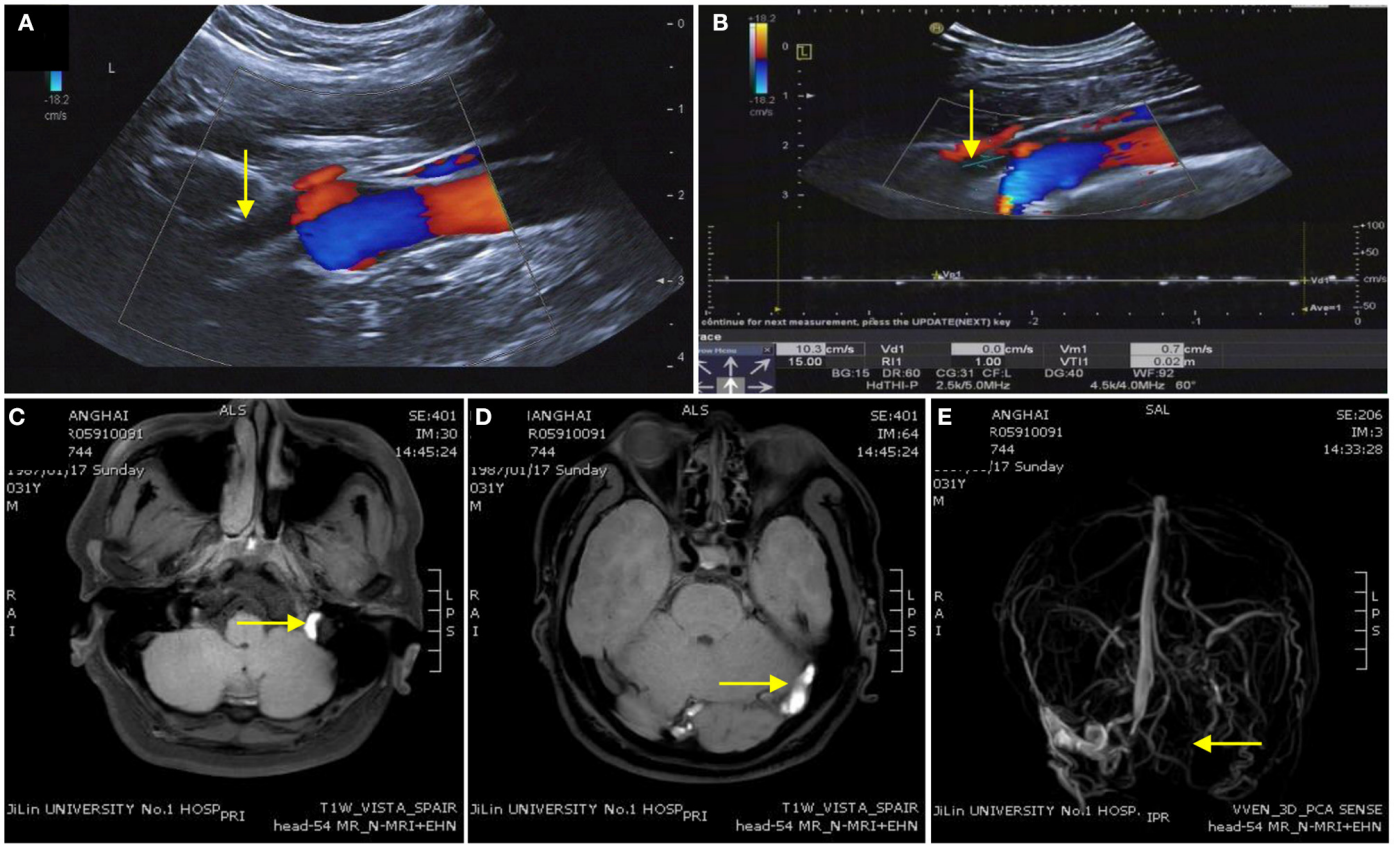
Imaging of a patient with cerebral venous sinus thrombosis. (A,B) Ultrasound image indicating the time-average-mean velocity of the internal jugular vein was extremely slow and unclear in the distant IJV-J3. (C,D) Enhanced magnetic resonance image showing the left sigmoid sinus, transverse sinus, and superior sagittal sinus thrombosis. (E) The superior sagittal sinus, left transverse sinus, left sigmoid sinus, and left IJV disappear on magnetic resonance venography.
